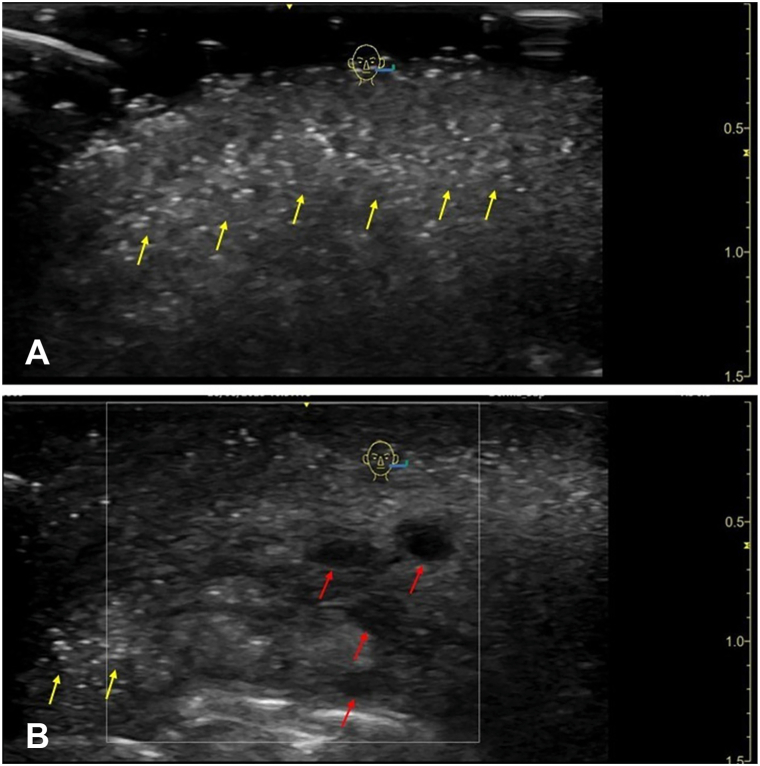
B-mode ultrasonography using a linear L8-12 transducer demonstrating: A, multiple bright hyperechoic foci with scattered comet-tail artifacts (*yellow arrows*) and mild posterior acoustic shadowing in the subcutaneous plane of the lips and perioral region, consistent with PMMA filler deposits; and (B) several anechoic pseudocysts (*red arrows*), consistent with the typical ultrasonographic appearance of hyaluronic acid fillers, and some comet-tail artifacts (*yellow arrows*) on the left. *PMMA*, Polymethyl methacrylate.
